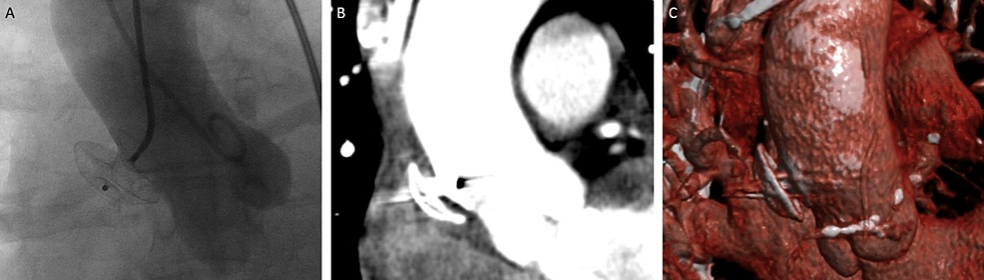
Post-Intervention Imaging. Aortography (A), CT scan (B), and 3D reconstruction (C) of the chest post-intervention showing no residual pseudoaneurysm or contrast extravasation.
